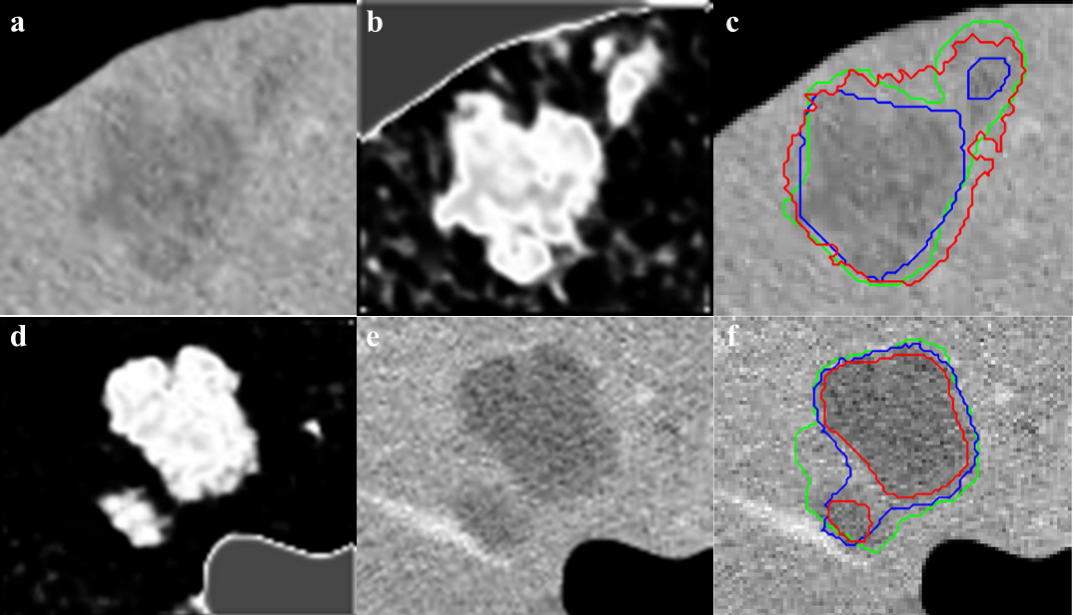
Cropped CT image with 22 mm and 7 mm tumors (a) and 32 and 9 mm tumors (d), initial CS-FCM clustering (b,e), radiologist segmentation (green outline) vs RW (red outline) (β=30) and RW_P_ (blue outline) (γ=0.003) (c,f)
